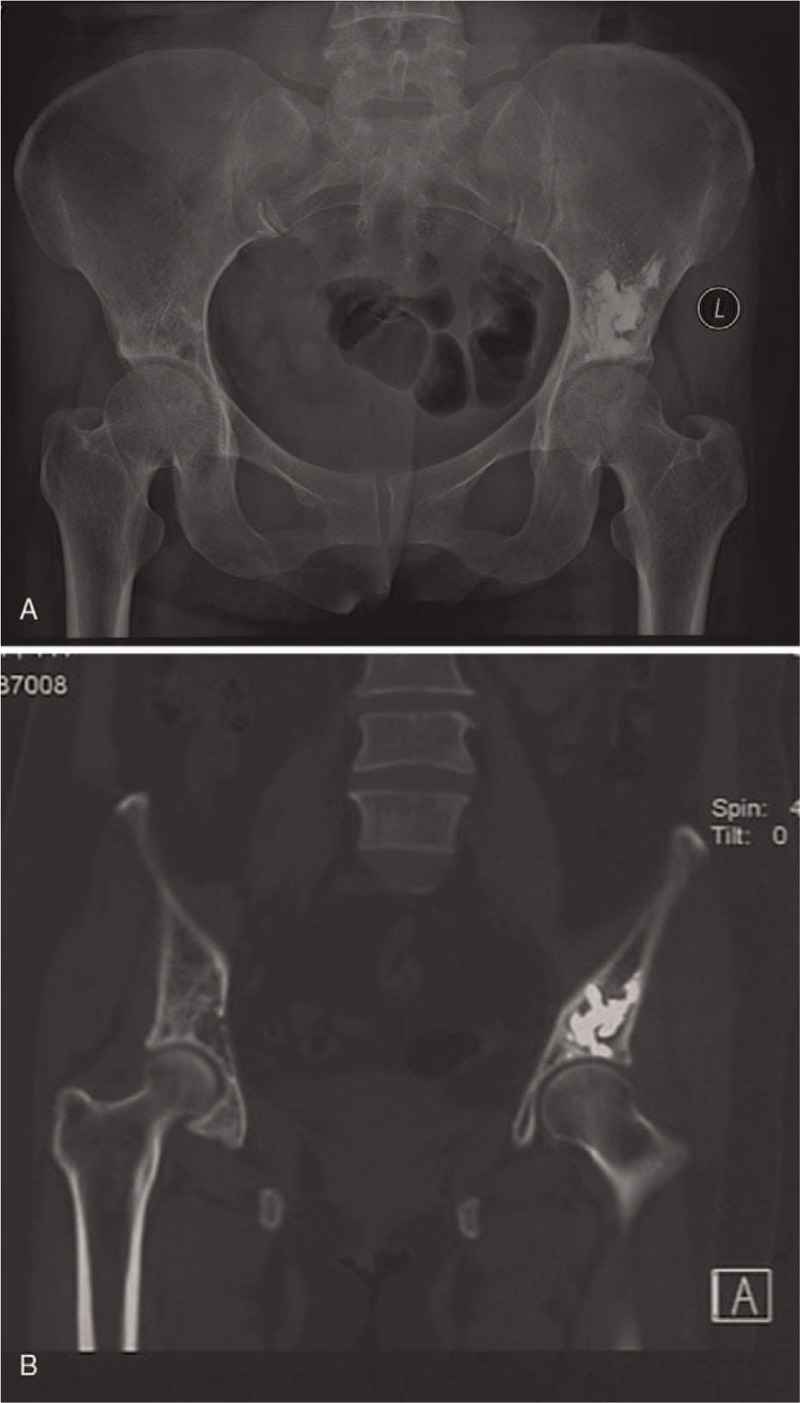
The x-ray film (A) and CT scan image (B) taken 24 months after isolated PA treatment did not reveal osteolysis or bone cement dislocation in the area of cementation (patient 2). PA = percutaneous acetabuloplasty.
